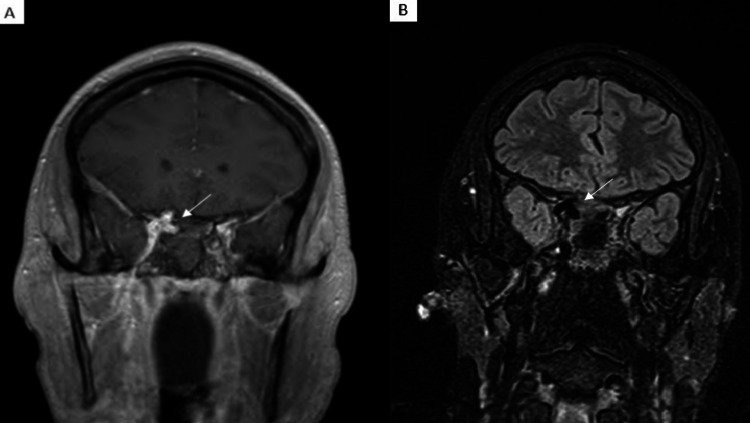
Right Cavernous Internal Carotid Artery Aneurysm Shown on Magnetic Resonance Imaging Coronal T1 post-contrast (A) and coronal T2 fluid-attenuated inversion recovery (FLAIR) sequence (B) magnetic resonance imaging demonstrates a right cavernous internal carotid artery aneurysm (white arrows). The left internal carotid artery flow-void is absent due to agenesis.
